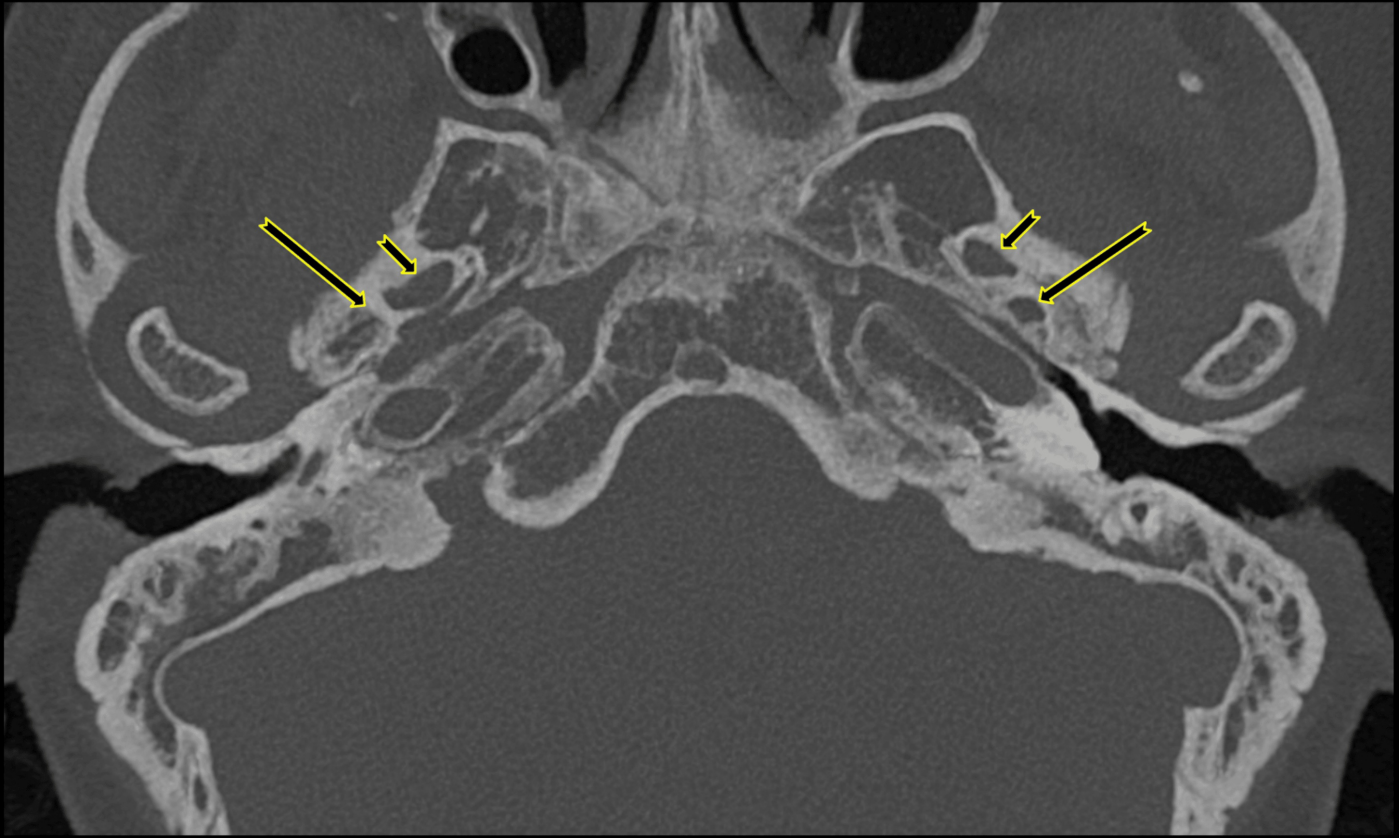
Axial CT reconstructions with maximum intensity projection of the temporal bones with the right PSA Normal foramen spinosum on the left and absent on the right at its expected location (long arrow); note the normal foramen ovale on both sides (short arrow). PSA: persistent stapedial artery
